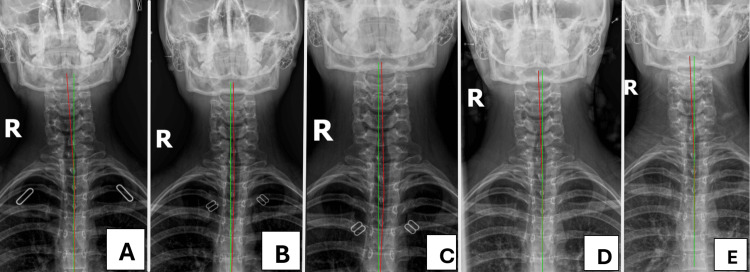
Anterior to posterior cervicothoracic radiographs from the initial examination (A) to follow-up examination (E). The patient’s right head translation improved following care and remained stable at long-term follow-up with only a slight return to baseline at panel E. The green line represents a vertical access line from the mid-thorax to the tip of the dens and the red line represents the best-fit line using the Risser-Ferguson analysis of the center of mass of the patient’s vertebral bodies.
